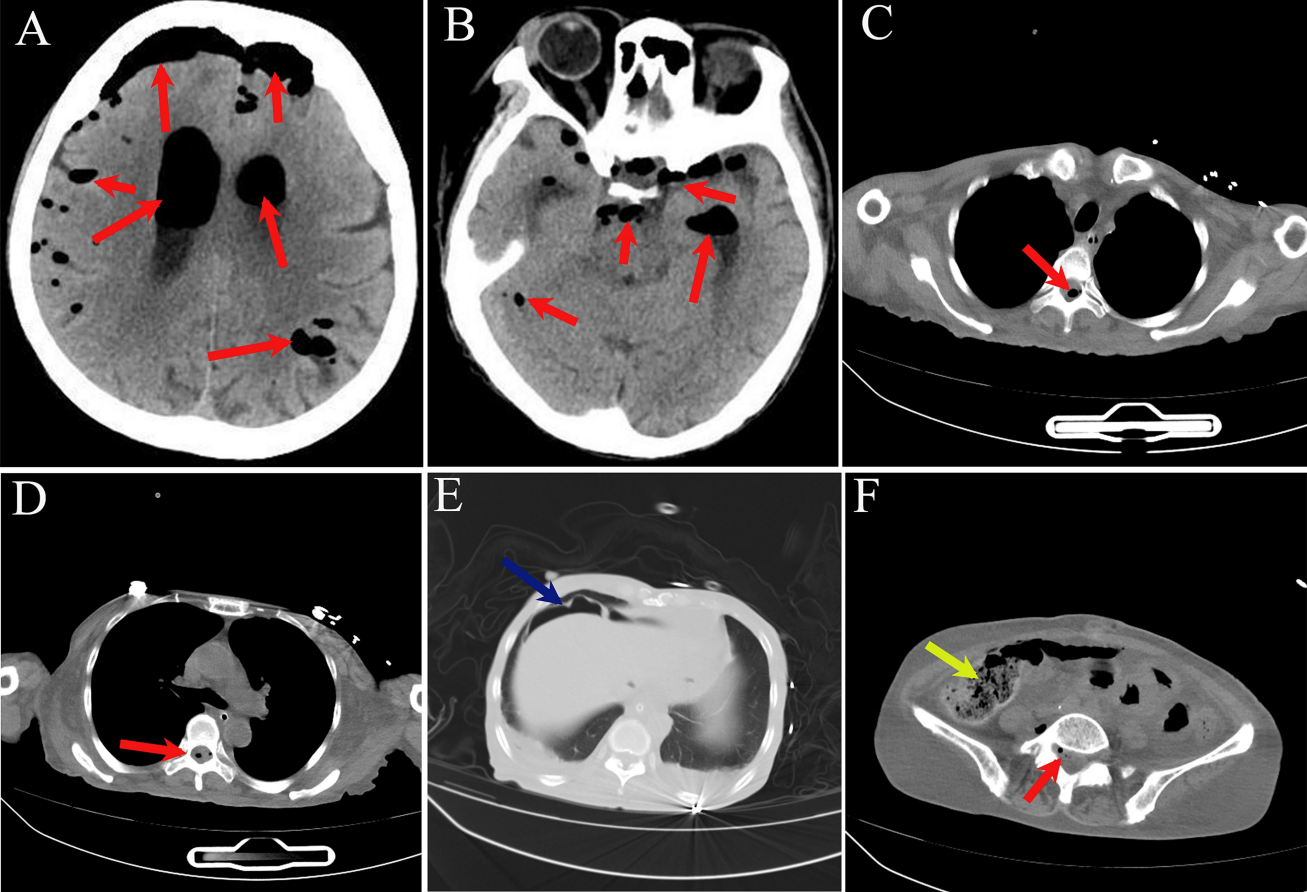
(A–F) CT of the head, thorax, and abdomen. Red arrows indicate pneumocephalus involving the intracranial space, ventricular system, and spinal canal; green arrows indicate free intraperitoneal air; yellow arrows indicate hyperdense fecalith.
